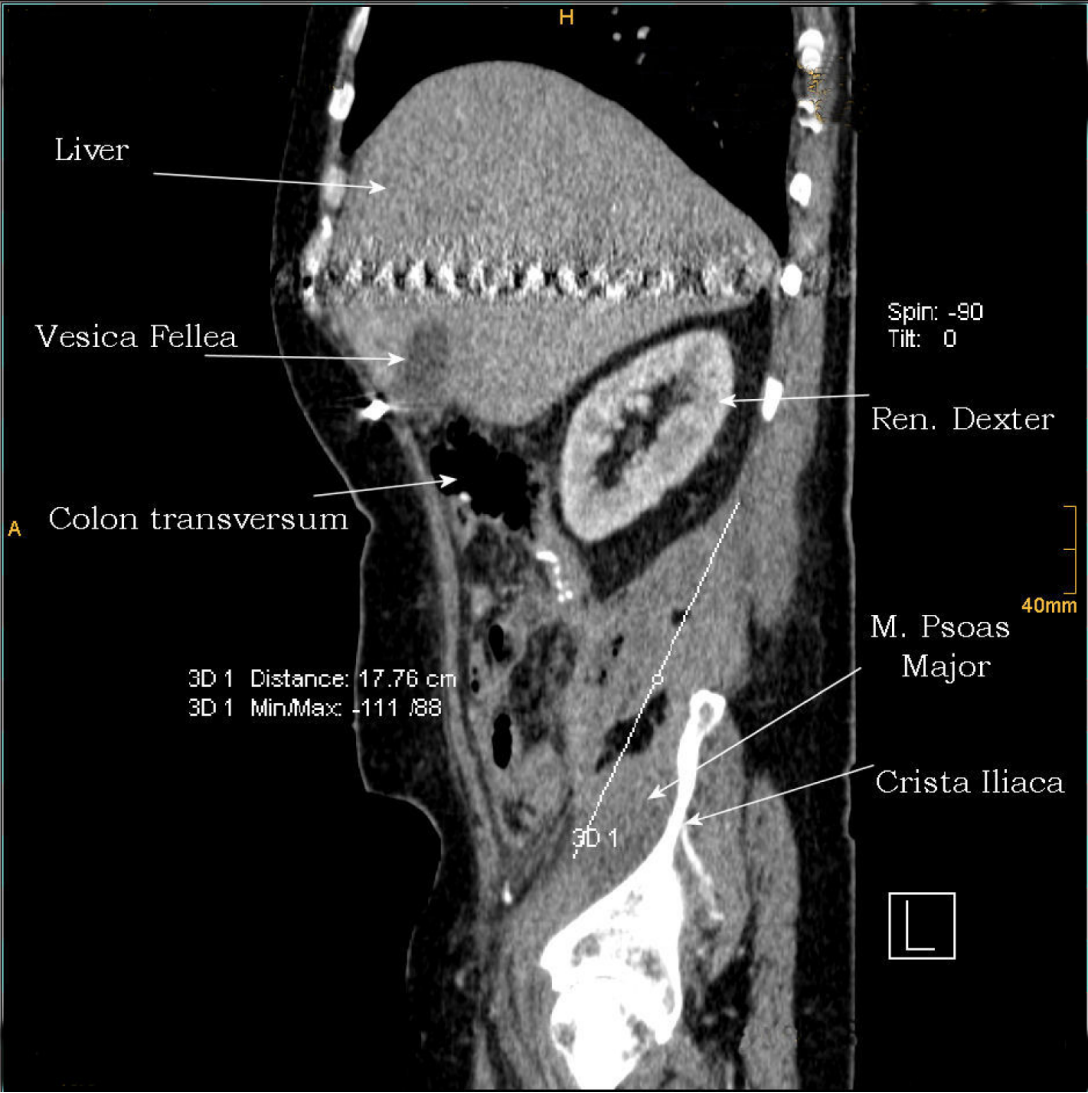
2^nd ^case. CT - sagittal plan of a large retroperitoneal hematoma - 17.76 cm.
